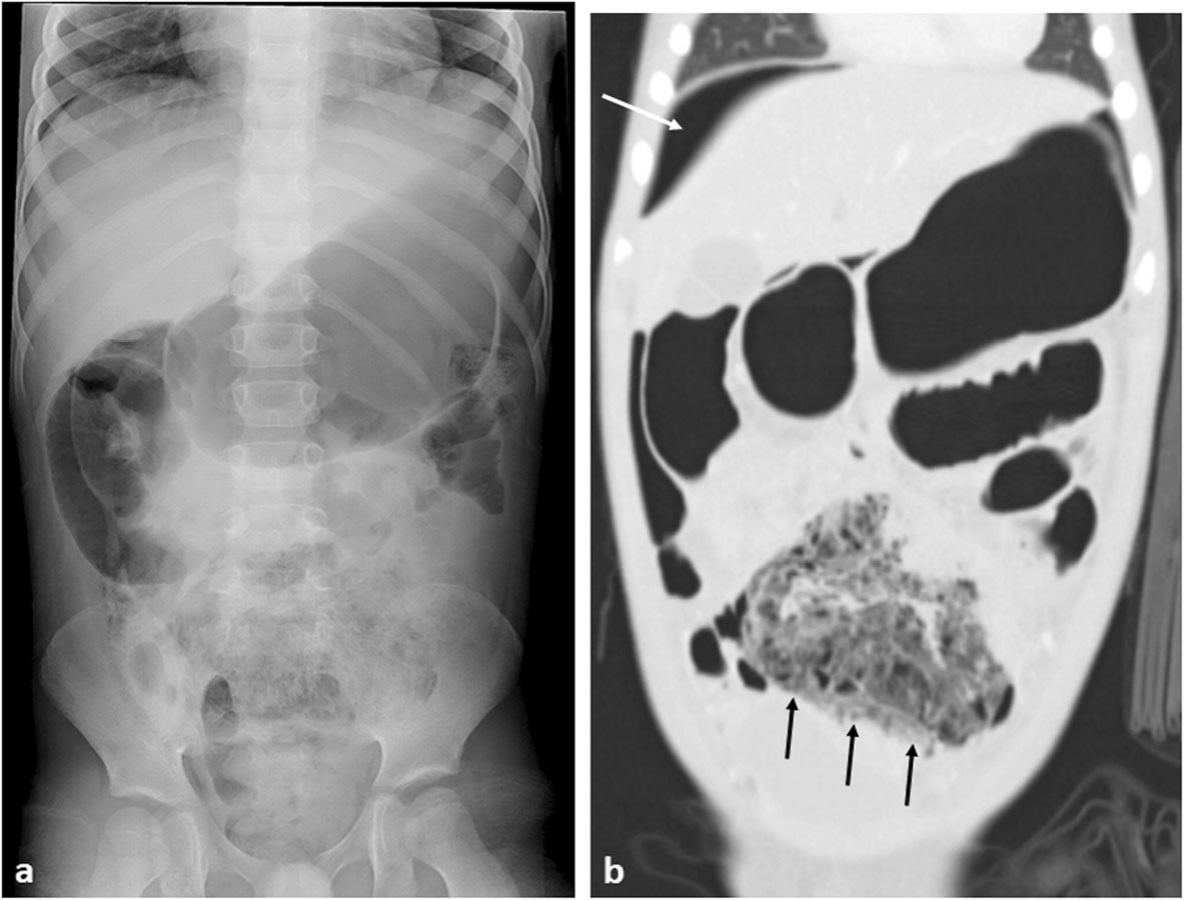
A 6-year-old boy with small bowel Burkitt’s lymphoma. a Abdominal plain radiograph demonstrates gastric and small bowel dilatation with a mottled gas appearance within the pelvis. b Coronal CT imaging with lung windows, demonstrates free intra-abdominal gas, best seen superior to the liver (white arrow), with proximal bowel obstruction and perforation of a thick-walled ileal mass (black arrow)
